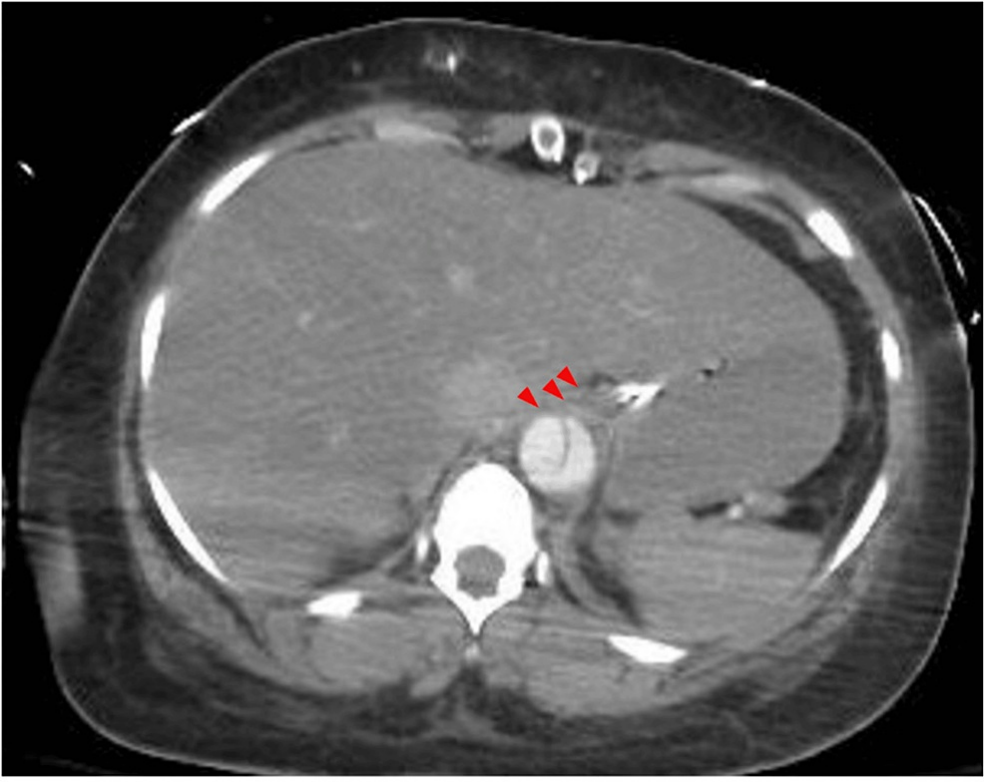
Computed tomography scan shows liver edema and ongoing dissection involving the celiac trunk Red arrows show celiac trunk
